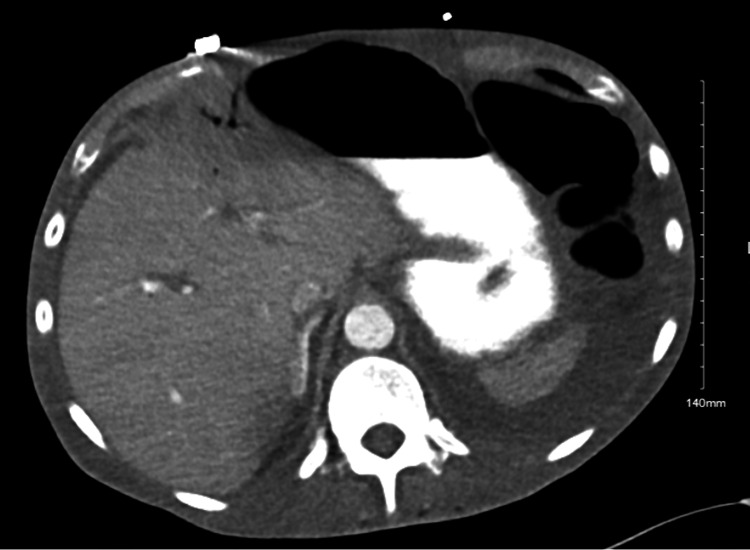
CT abdomen with portal venous gas
